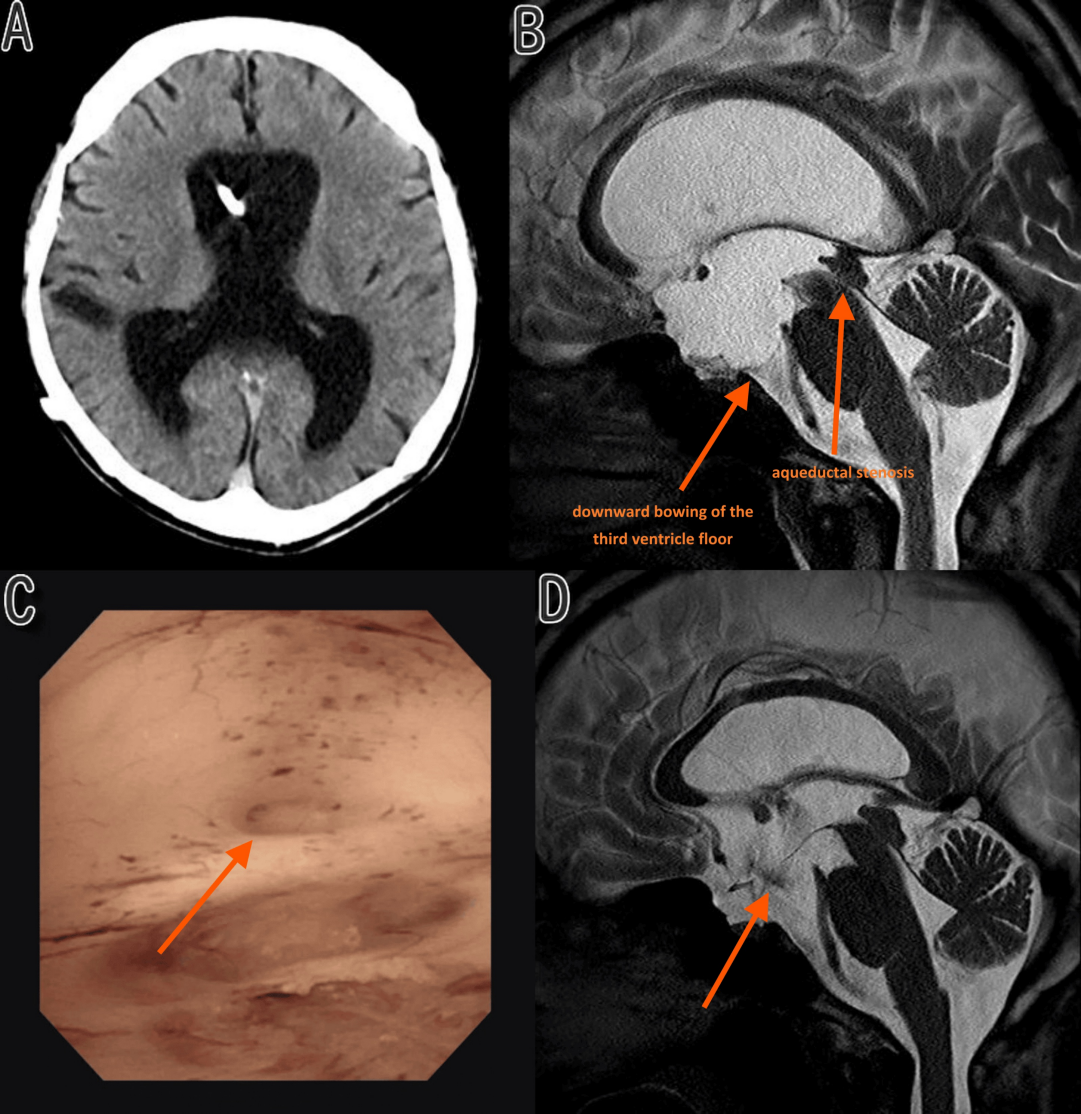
Case 2: (A) Preoperative CT (lateral ventricle enlargement due to shunt obstruction). (B) Preoperative MRI T2WI. (C) Intraoperative endoscopy showing scar stenosis. (D) Postoperative MRI on day 6 showing stoma patency CT: computed tomography; MRI: magnetic resonance imaging; T2WI: T2-weighted imaging
